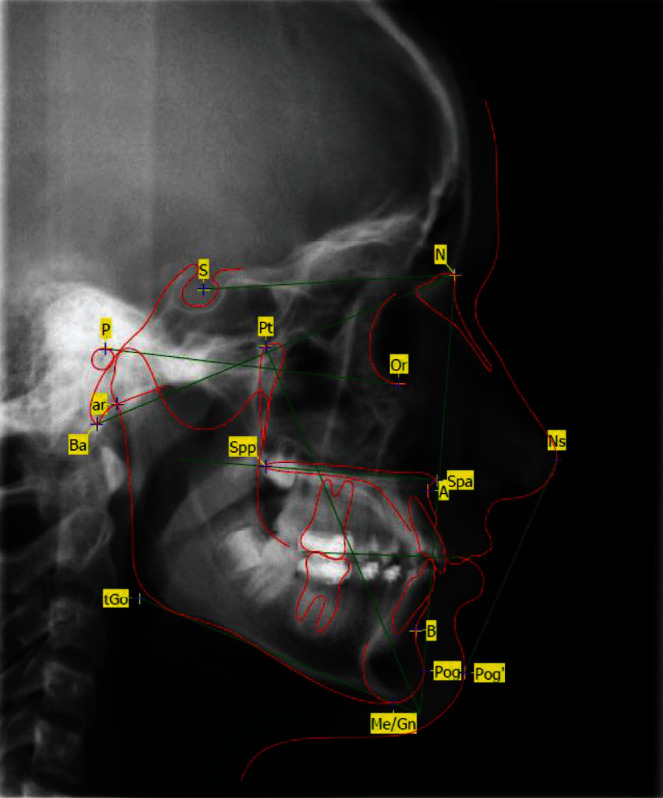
Lateral cephalometric radiograph with evaluation 48 months postoperative (end of treatment); for abbreviations, see also Table 1.
